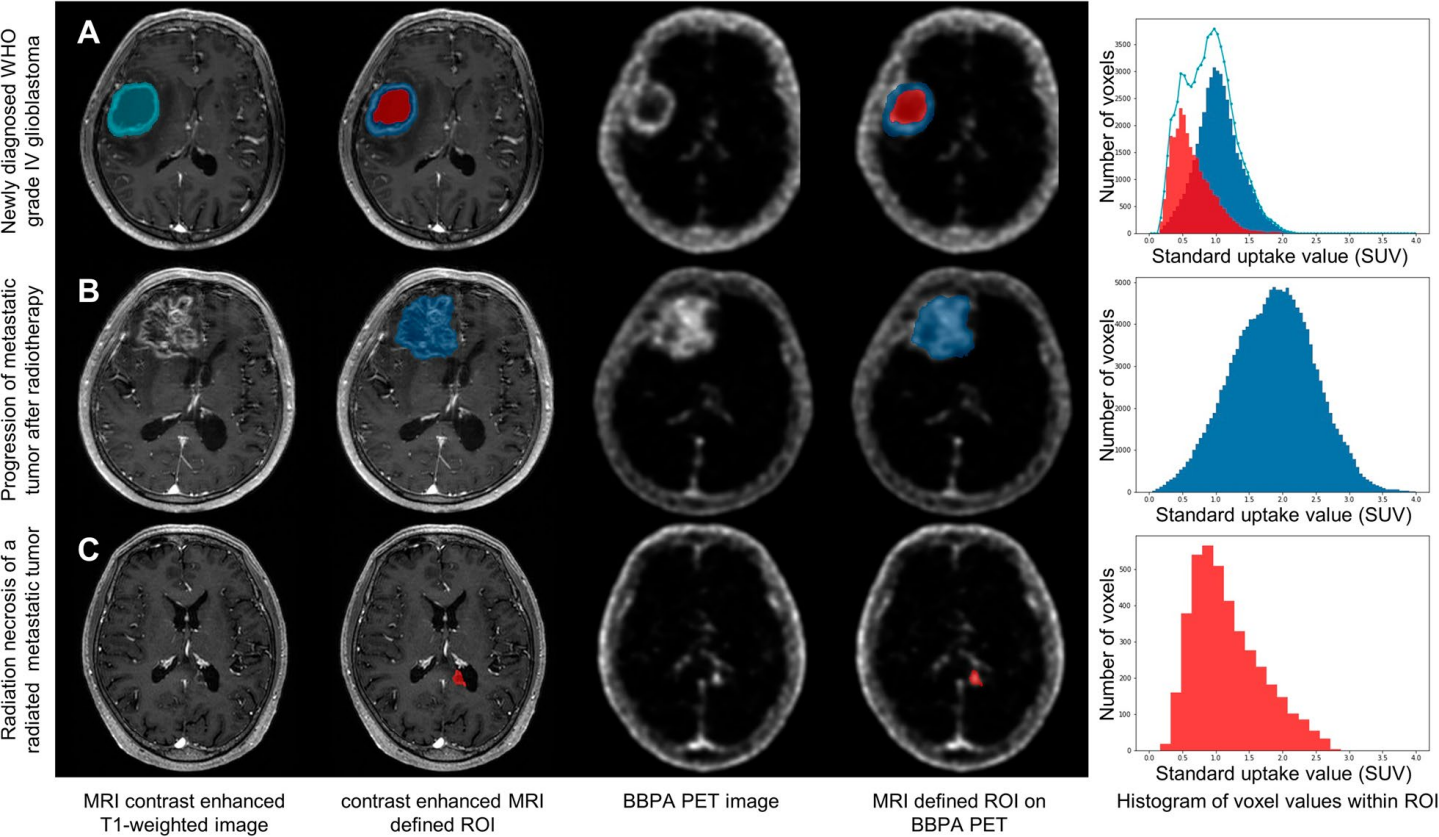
[^18^F]BBPA histogram for differential diagnosis in clinical scenario. A A 62/M patient displayed right frontal lesion with ring-like contrast enhanced on MRI, and the whole lesion (light blue area), contrast enhanced area (blue area) and non-contrast enhanced area (red area) were semi-automatically defined. The contrast enhanced (blue) area exhibited a BBPA uptake similar to normal distribution which is in accordance with tumor characteristics, while the central (red) region revealed a positive skewed [^18^F]BBPA activity that is corresponding to non-neoplastic lesion. The whole tumor displayed a dual-peaked histogram pattern (light blue line) that can be divided into two single peaks on the separate segmentations (red and blue area), and this metabolic characteristics was suggestive of glioblastoma. B A 71/F patient exhibited right frontal metastatic breast cancer and received cranial radiotherapy and tyrosine kinase inhibitor. Four months after treatment, the tumor was considered to have treatment response thanks to the slightly improved volume effect on MRI. However, the lesion displayed increased symmetric [^18^F]BBPA activity, suggesting there was remaining active tumor. The patient continued tyrosine kinase inhibitor treatment, and six months after [^18^F]BBPA PET, the patient progressed clinically and radiologically. C A 63/M patient with periventricular metastatic lung cancer received radiotherapy and achieved completed response on MRI. Fifteen months after radiotherapy, the patient developed regional abnormal signal on MRI that was initially considered as tumor recurrence. However, the lesion exhibited positive skewed [^18^F]BBPA distribution that was suggestive of non-neoplasms, and the lesion remained radiologically stable at 1-year follow-up (without anti-tumor treatment)
